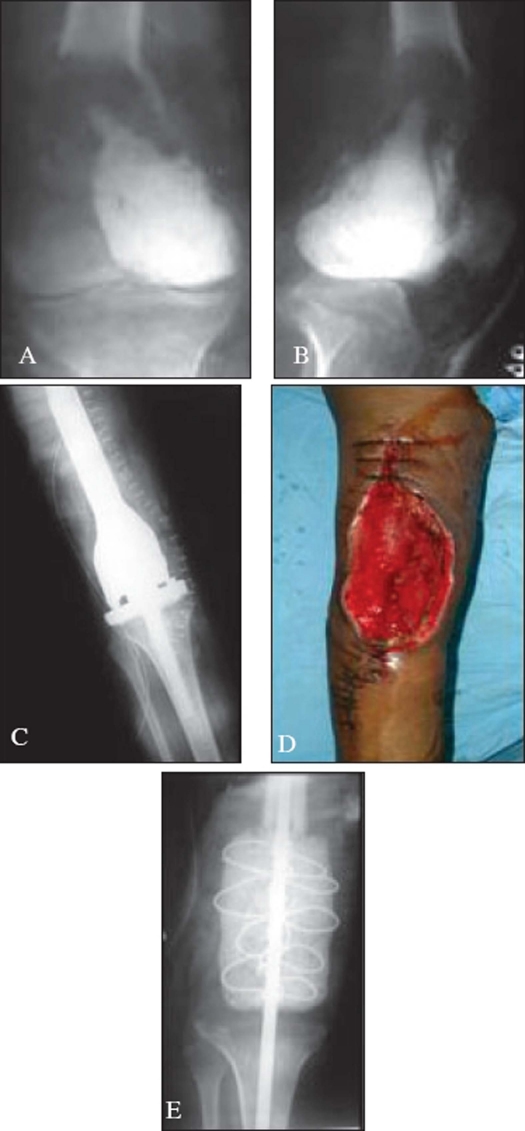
A), B) X-rays (A.P.) and lateral) of knee shows a recurrent GCT distal end femur in a 25-year-old female. C) Postoperative x-ray of the same patient showing tumor excision and endoprosthesis insertion. D) Post operative picture of the same patient with coverage problems of the wound. E) 5 month post op picture of the same patient showing removal of prosthesis and cement spacer insertion. Patient underwent ampution after 13 months due to uncontrolled infection
